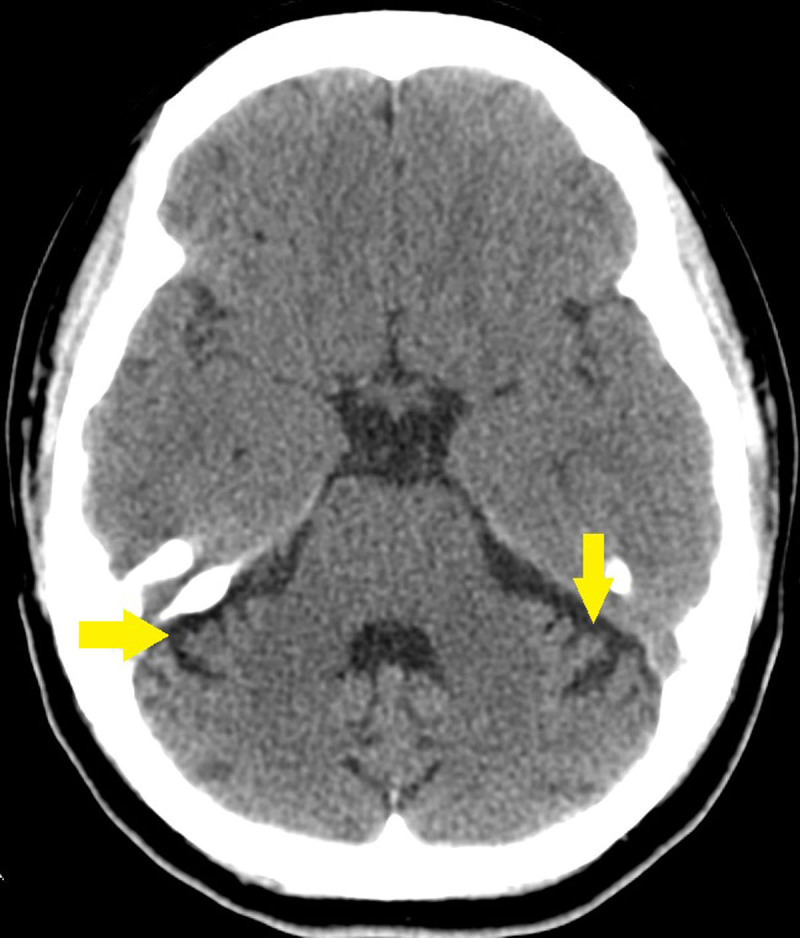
Unenhanced CT axial image showing atrophy of the cerebellar folia (yellow arrows). Note the normal appearance of pons.
